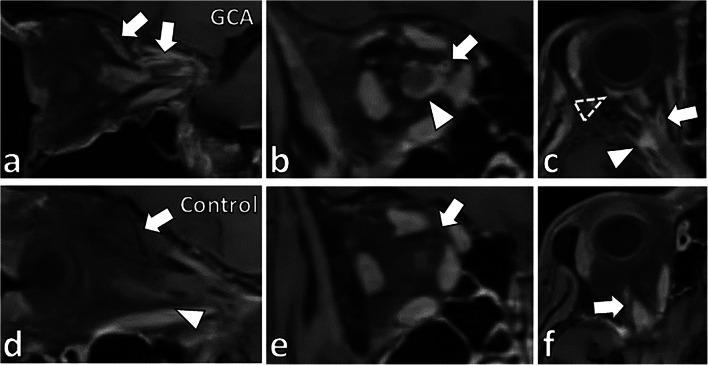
Vessel wall enhancement of the ophthalmic artery and its branches in a patient diagnosed with GCA. In a patient diagnosed with GCA, (a) sagittal, (b) coronal, and (c) axial BB-MRI images show concentric vessel wall thickening and enhancement of the right ophthalmic artery (a–c, arrows). There was also optic nerve sheath enhancement (b–c, arrowheads) and partial rim enhancement posterior to the globe (c, dashed arrowhead). In an age-matched control case, (d) sagittal, (e) coronal, and (f) axial BB-MRI show no vessel wall thickening or enhancement of the ophthalmic artery (d–f, arrows). No pathologic enhancement of the optic nerve sheath (d, arrowhead) or other orbital structures was identified
